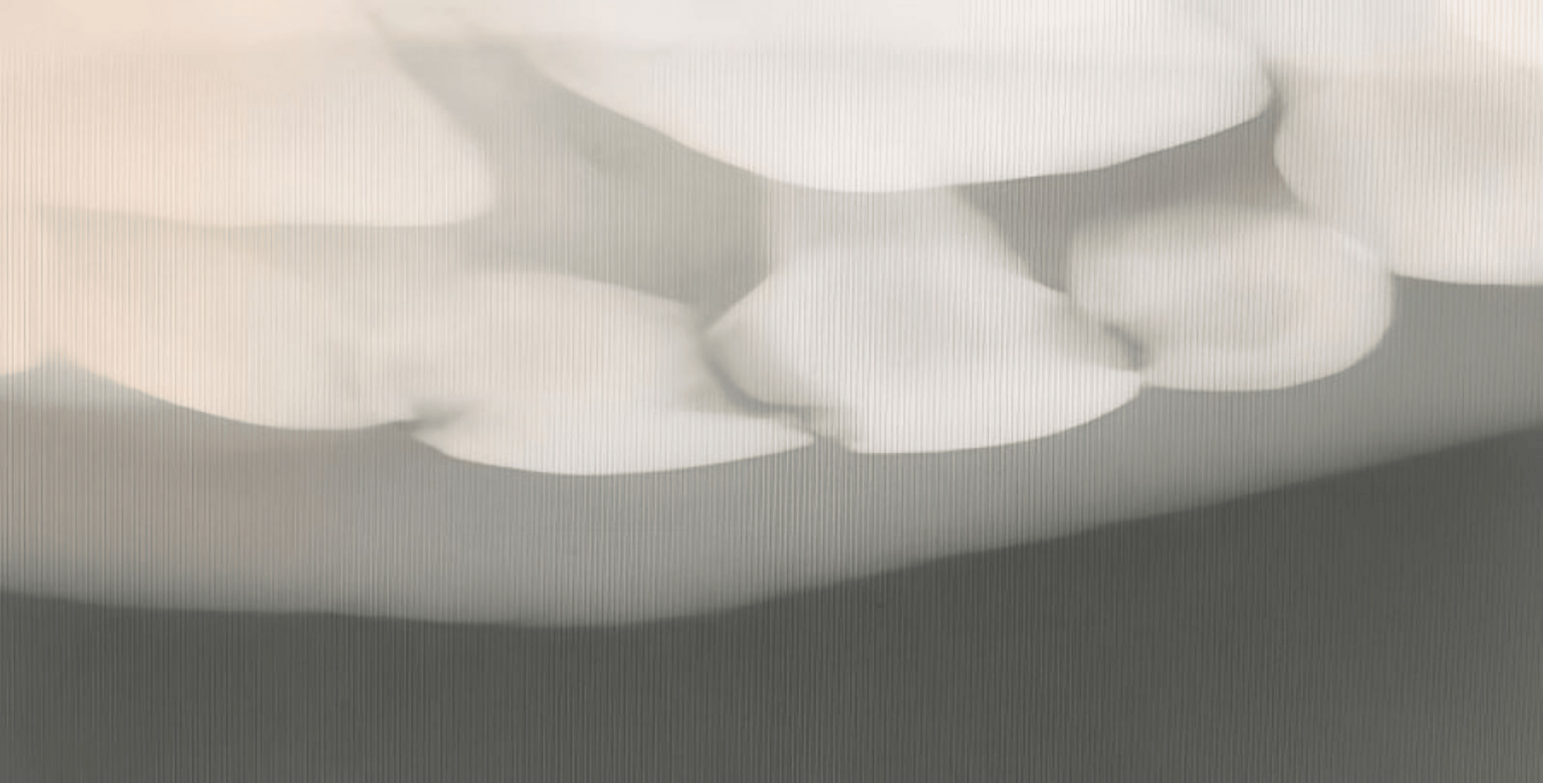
Intraoral periapical radiograph (IOPA) showing the right maxillary anterior region of the jaw. IOPA: intraoral periapical radiograph
